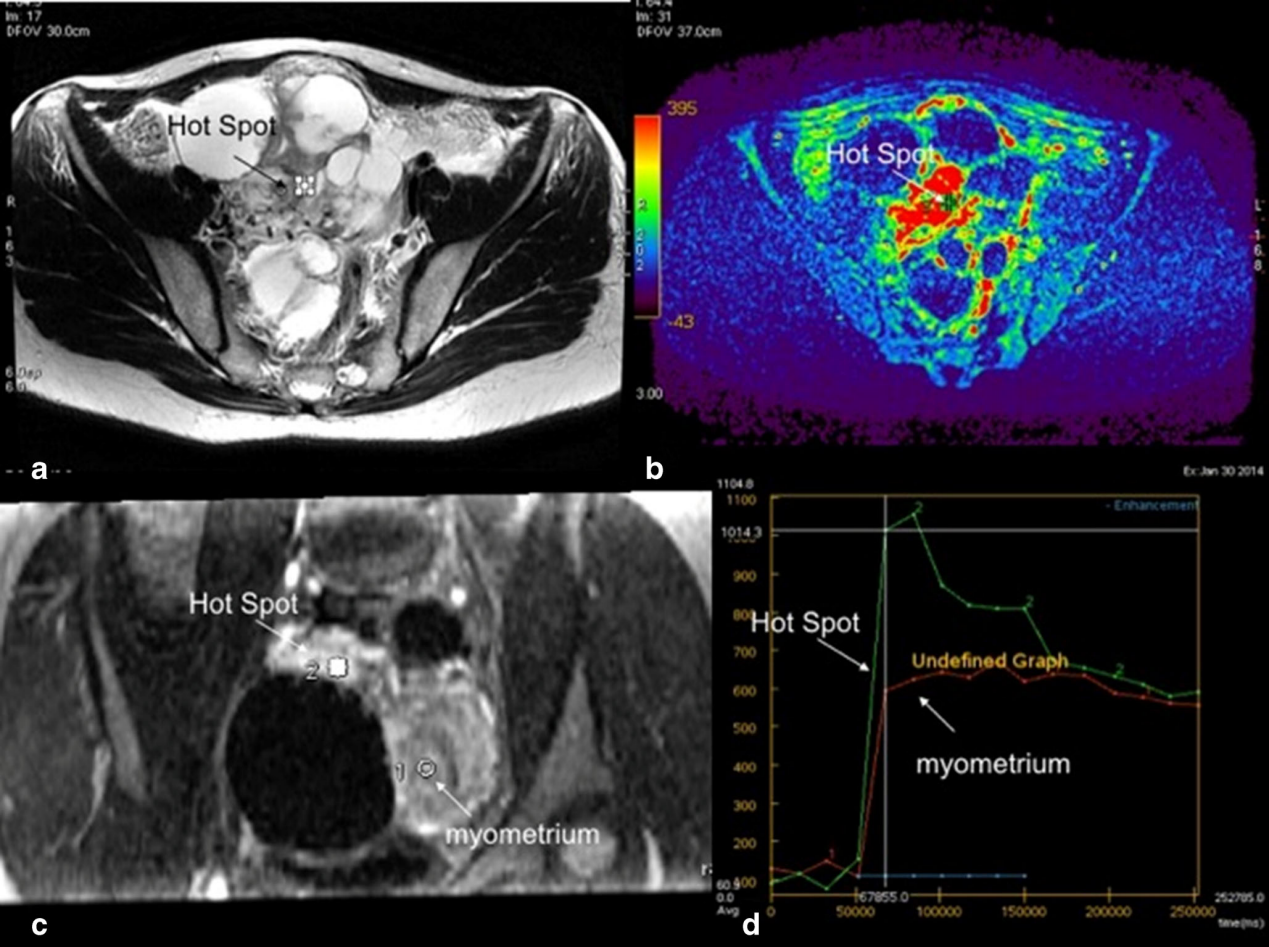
Technical assessment of DCE MR imaging in complex adnexal masses. This example shows a complex right ovarian mass with a solid component in intermediate T2W signal (a) that heterogeneously enhances after gadolinium injection. Parametric map (maximal slope) helps to determine the most suspicious location (hot spot) where the region of interest should be placed to build the time–intensity curve (b). To compare this curve with the myometrial curve, 3D T1W sequence must be reformatted in the coronal plane to place the two ROI (solid component and external myometrium) (c). Comparison of time–intensity curves shows that the solid component enhances according to a time–intensity curve type 3 (curve steeper than that of myometrium)
